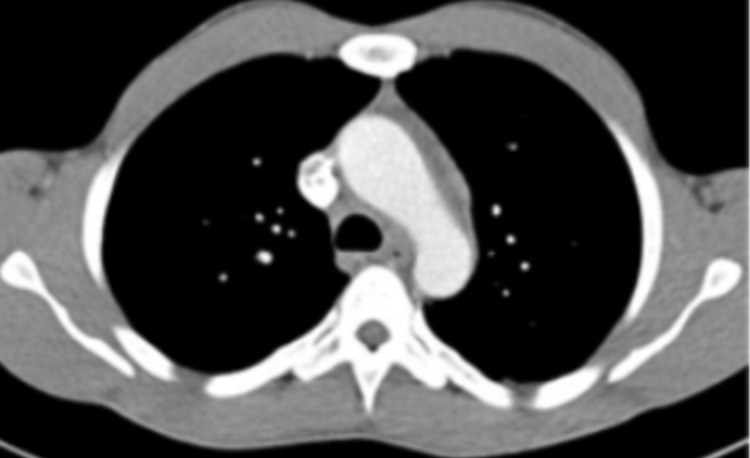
Chest CT scan. Chest CT scan showing bilateral diffuse subpleural and perifissural micronodules.
